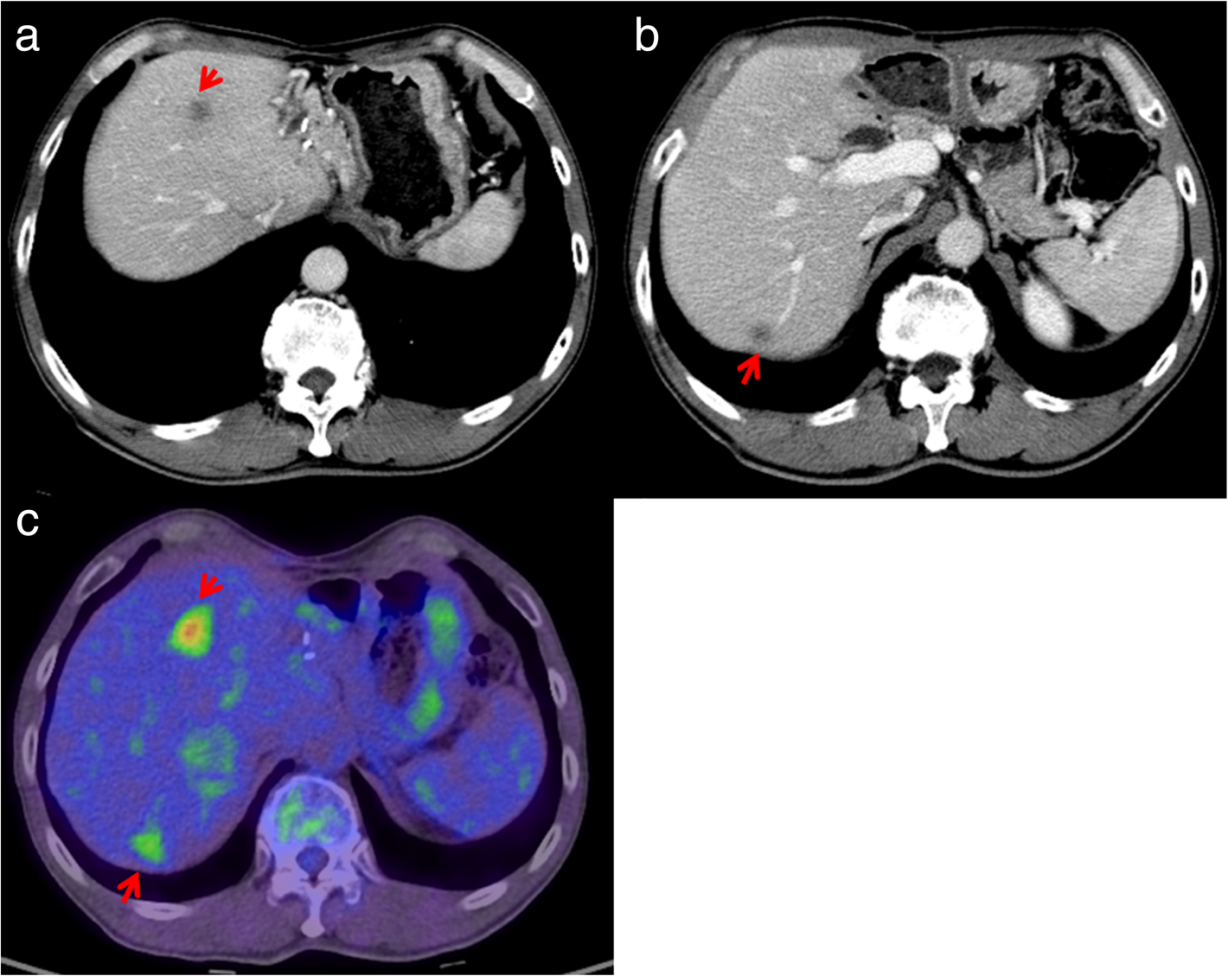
CT scan shows hypovascularization in hepatic S4/S8 (a) and S7 (b). c On PET-CT, abnormal FDG uptake is observed in both hepatic tumors (arrows)
